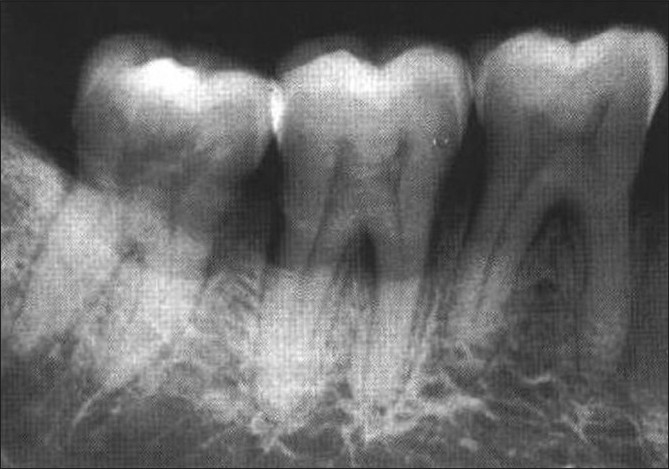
Radiograph of experimental site at 3 months
